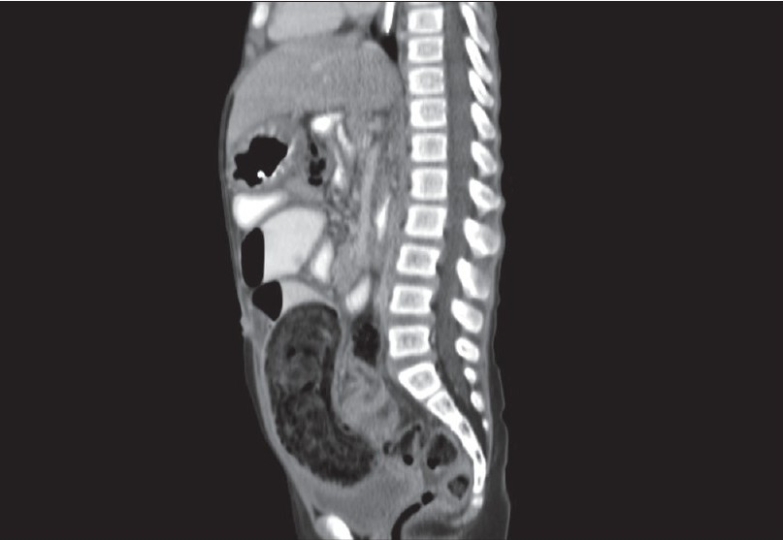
Sagittal view on CT with bezoar
